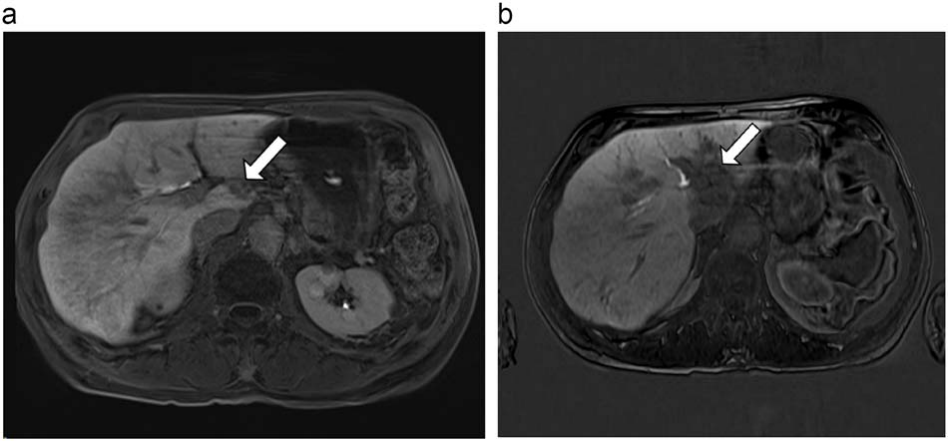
(a) Pre-ablation MRI demonstrating 15 mm lesion in caudate lobe of liver (image degraded by motion artifact). (b) Post-ablation MRI (20 days postoperatively) demonstrating 35 mm complete ablation of caudate lesion (image degraded by motion artifact).
